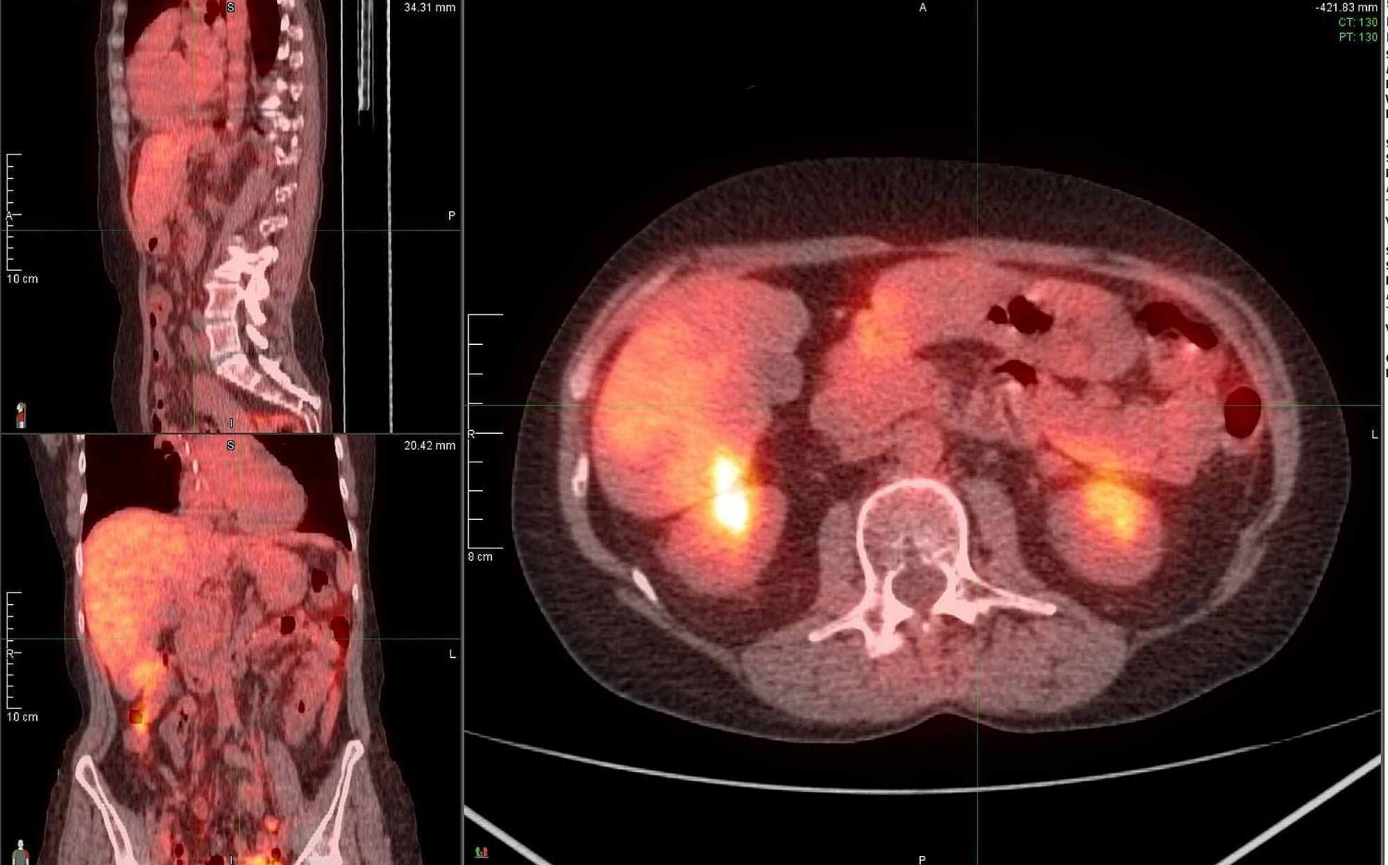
PET-CT demonstrating complete remission two months after starting treatment with pembrolizumab
